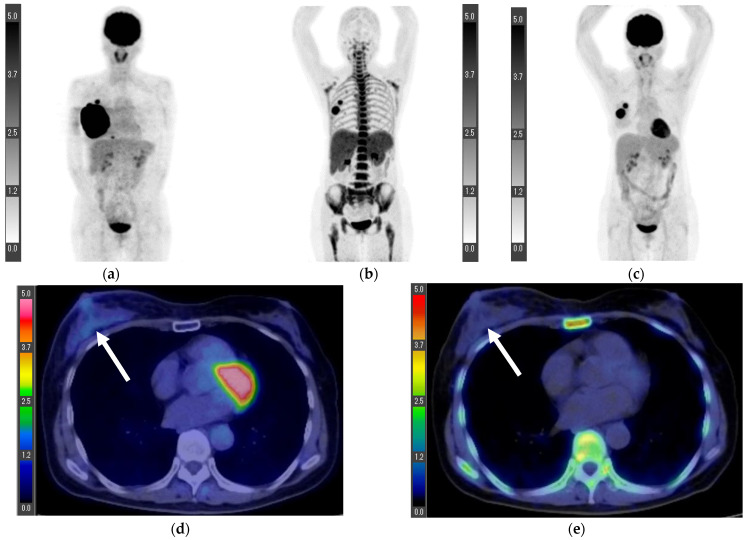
Maximum intensity projection (MIP) of ^18^F-FDG PET (a), MIP of ^18^F-FLT PET 7 months after chemotherapy (b), and MIP of ^18^F-FDG PET 4 days after the ^18^F-FLT PET (c). ^18^F-FDG accumulation increased in the right breast following chemotherapy ((e), arrow), whereas ^18^F-FLT accumulation was not evident ((d), arrow). Patient 7 (Figure 5).
